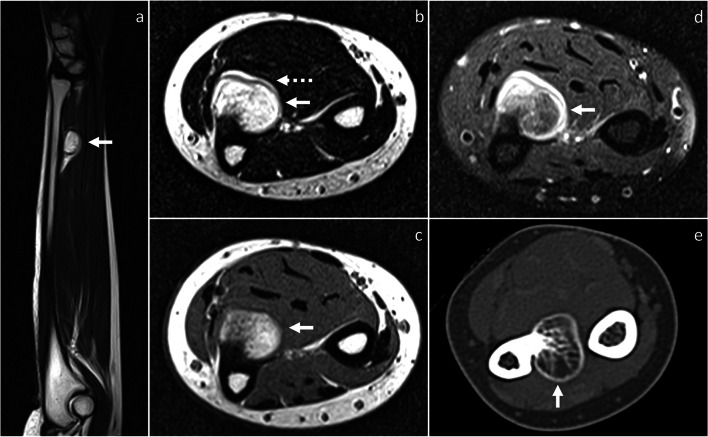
Recurred BPOP arising from the distal ulnar diaphysis (same patient shown in Fig. 4). Three years after surgery, BPOP recurrence presents as a cortex-based completely ossified mass, which is contiguous to the palmar-radial aspect of the ulna. On MRI, the ossified component of the mass is hyperintense on both T2-weighted (a, b) and T1-weighted (c) sequences. After contrast administration, contrast enhancement is noted on fat-saturated T1-weighted sequence (d). No cortical breakthrough is seen on CT (e). White arrow points at BPOP in all images. Dashed arrow points at the outermost cartilaginous layer of BPOP in (b)
